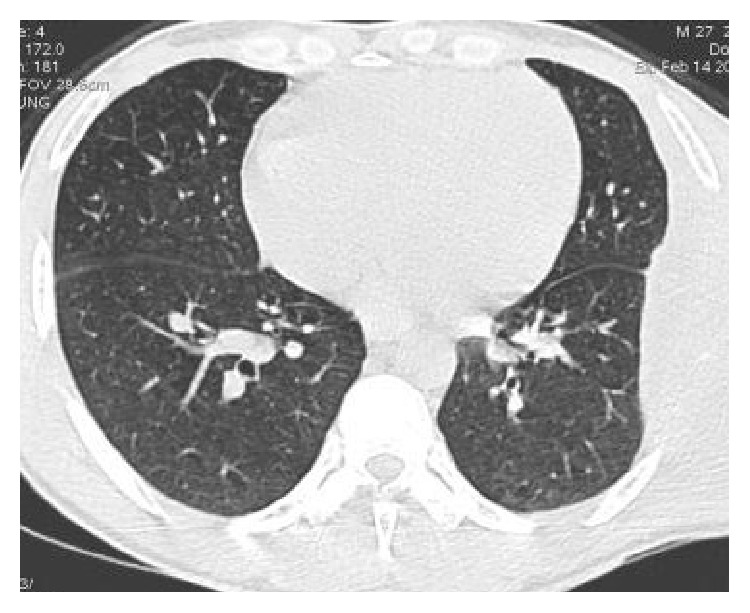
Axial Computed Tomography image at lesion level in lung window showing no evidence of lung parenchymal lesion.
